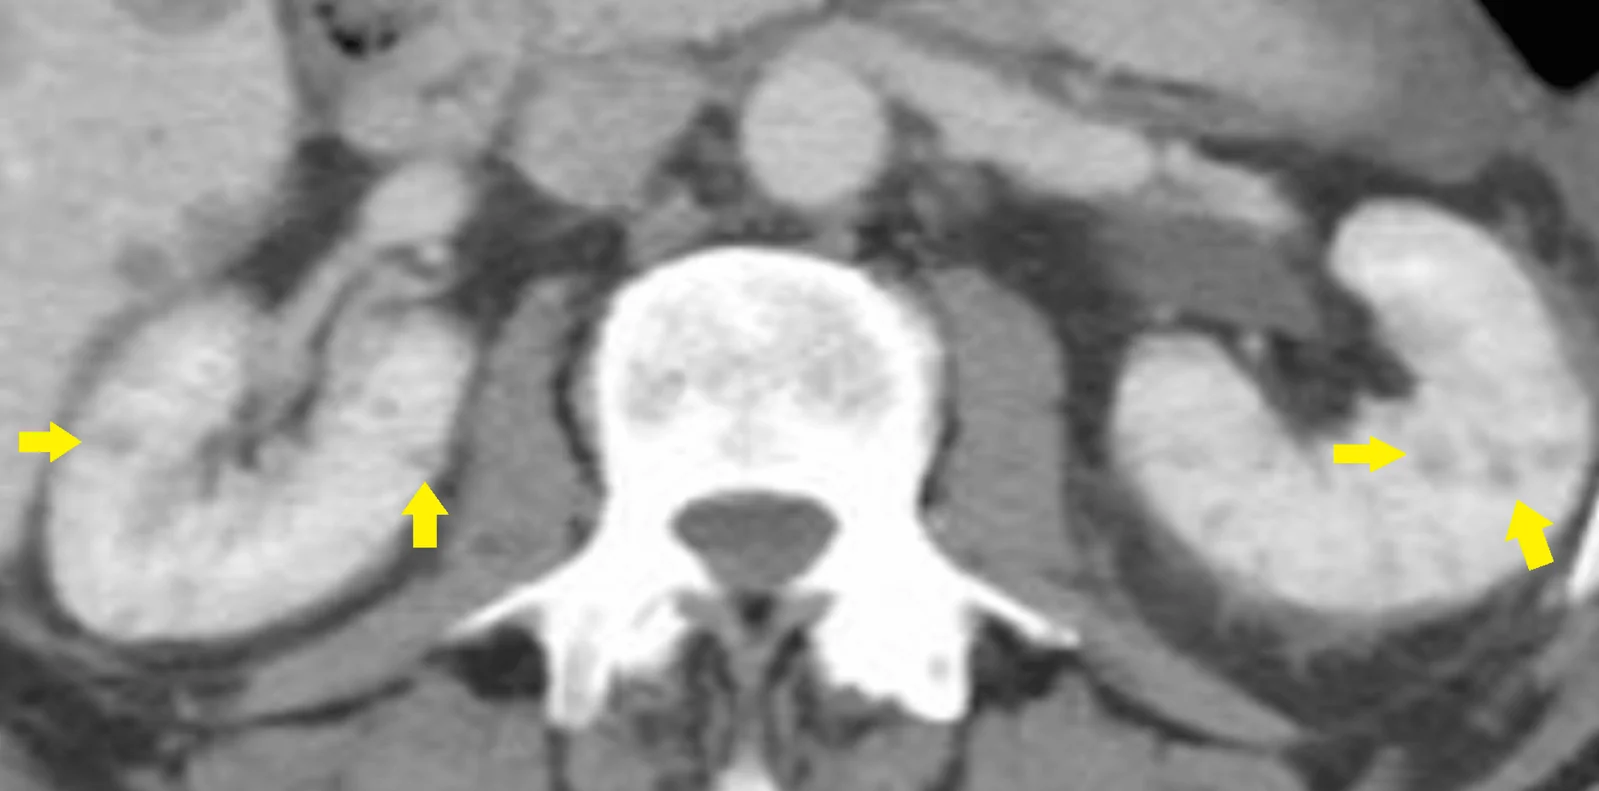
CT scan of the abdomen demonstrating multiple bilateral renal cortical hypodensities The yellow arrows point to multiple bilateral renal cortical hypodensities.
